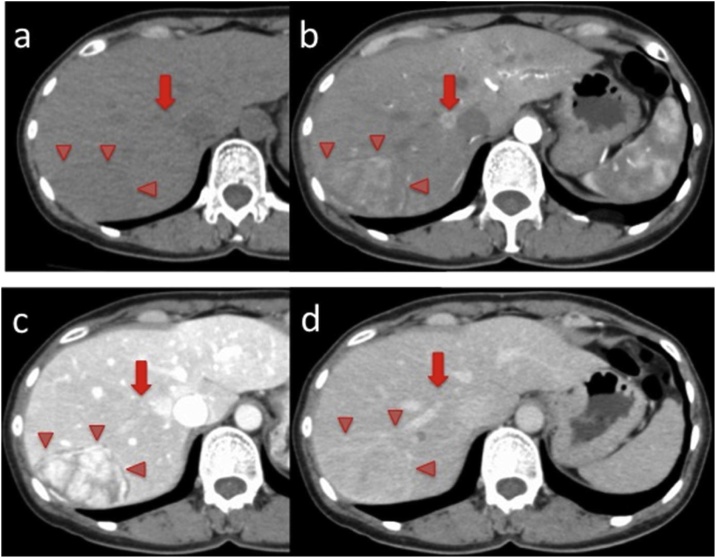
Plain and enhanced CT findings. A 61 mm tumor in the posterior section was observed as (a) a low density area in the plain phase, (b) a slightly high density area in the artery phase, (c) a strong high density area in the portal phase, and (d) an isodensity area in venous phase. A 10 mm tumor at the root of right hepatic vein was observed as (a) a low density area in the plain phase, (b) a high density area in the artery phase, (c) a high density area in the portal phase, and (d) an isodensity area in the venous phase.
